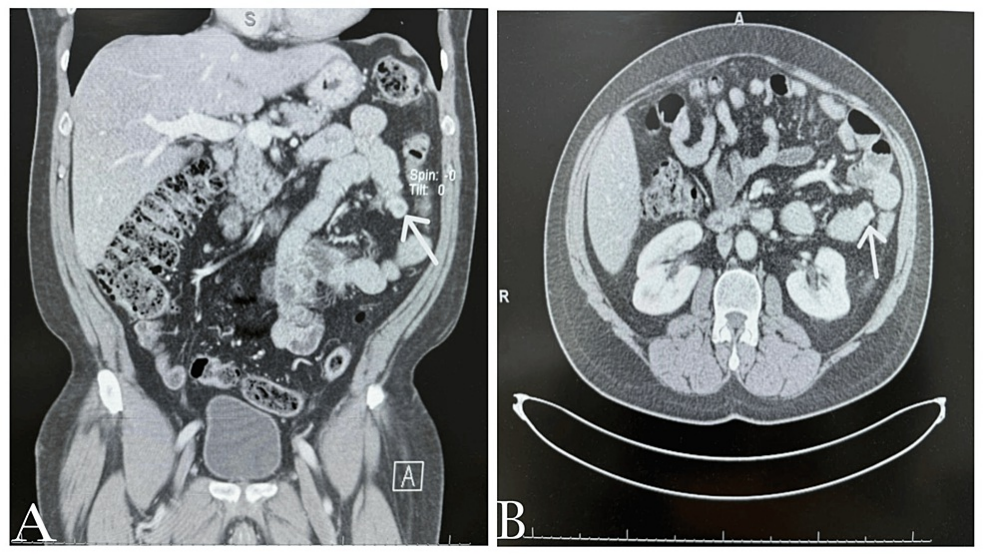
Different views of the tumor in the abdominal CT scan. A) Small tumor (indicated by arrow) in the wall of the proximal jejunum, measuring 2 × 1.5 × 2 cm, in the coronal view of the CT scan.
